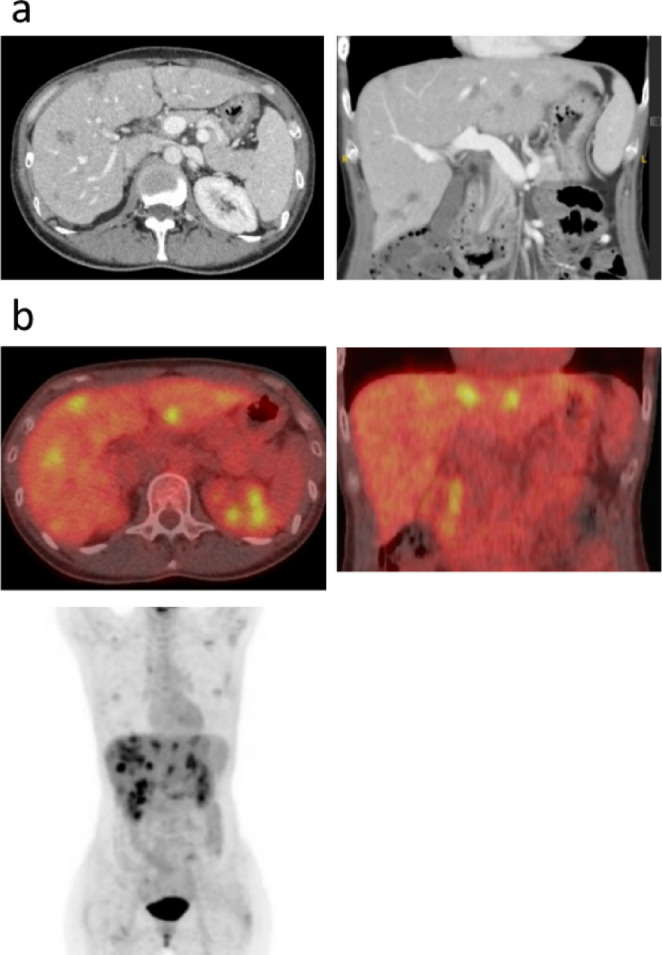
Computed tomography (CT) and fluorine-18 fluorodeoxyglucose positron emission tomography (FDG-PET) images before liver transplantation. Although this patient had pathological positive lymph node metastasis at Station 12b, no perihepatic lymph node swelling was identified on CT or PET
